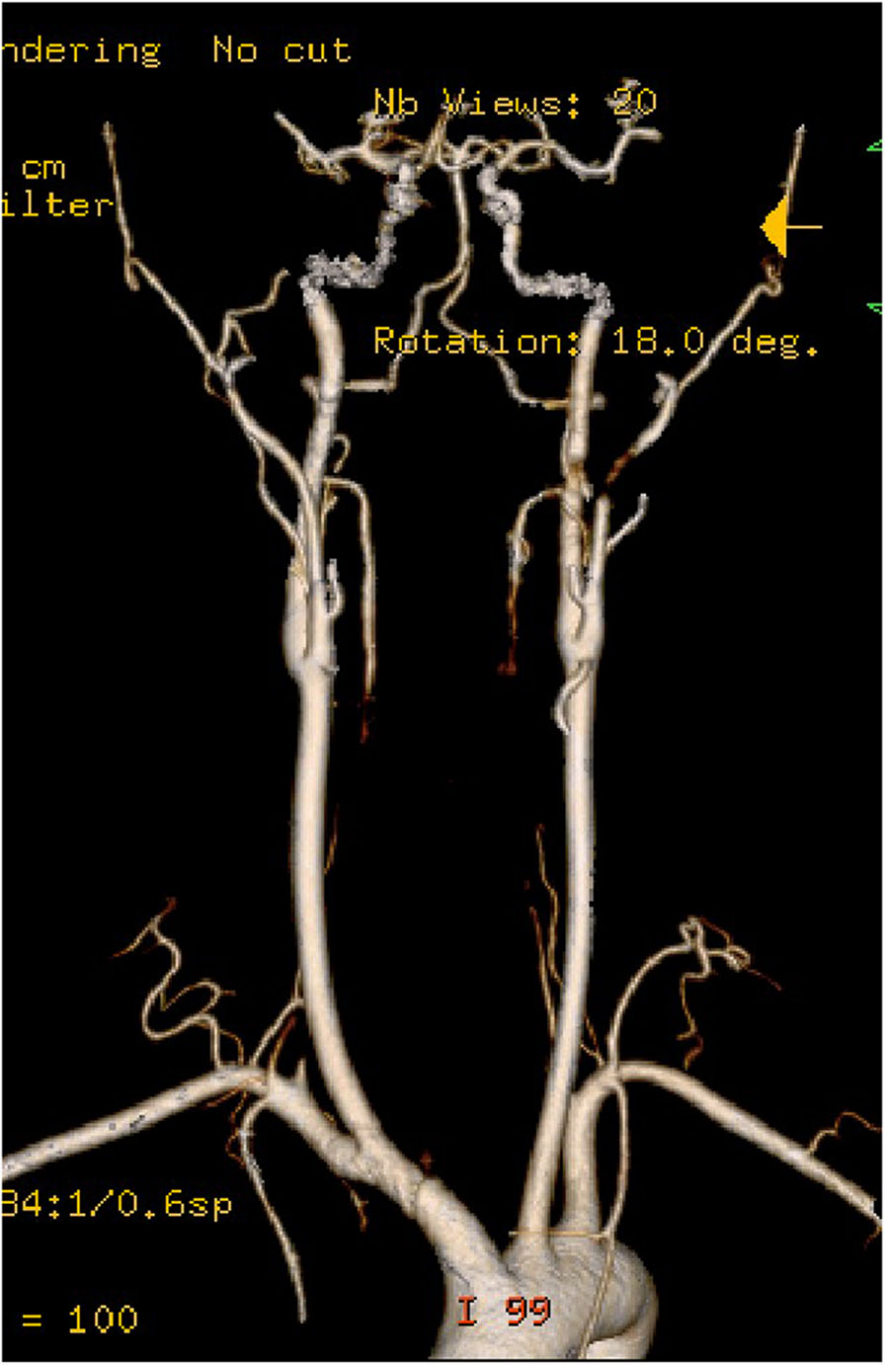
CTA demonstrated the narrowing of bilateral vertebral arteries consistent with the spinal injury levels
